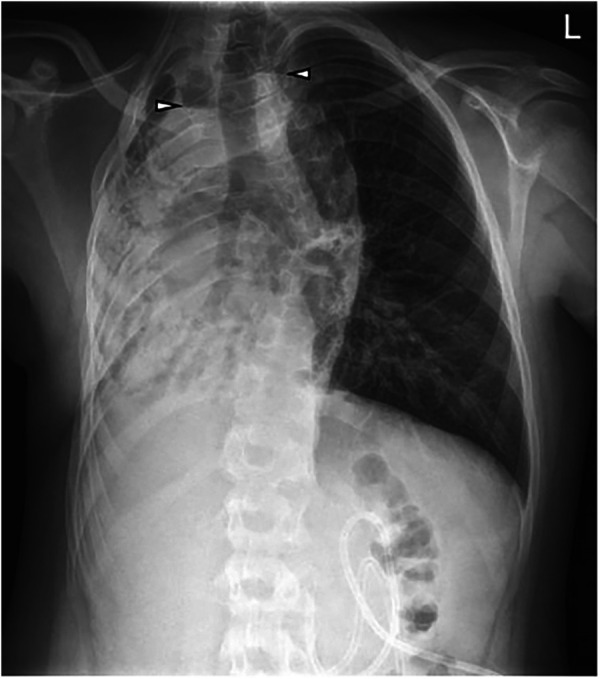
Initial chest x-ray at the age of 13 years 6 months: G-tube in the stomach. Volume loss and opacification of the right lung with focal air-filled bronchiectasis. Two areas with air-fluid levels in the upper mediastinum and right lung (arrowheads). Compensatory hyperinflated left lung.
